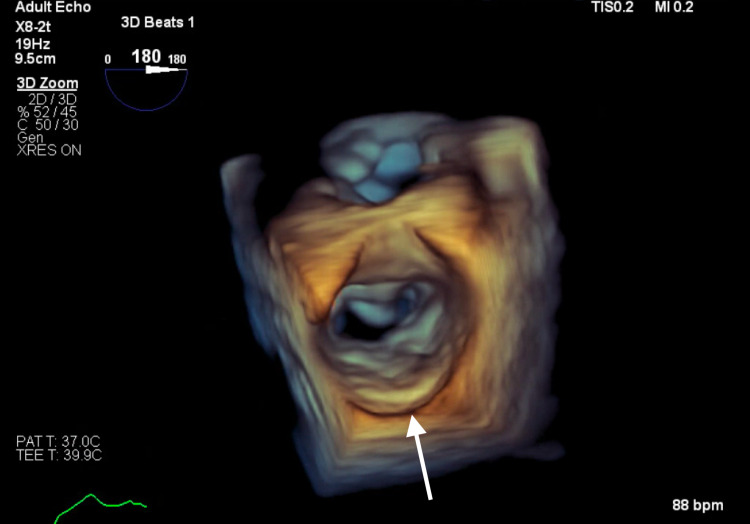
Transesophageal echocardiogram 3D surgeon view showing calcification of the left atrial posterior wall.
